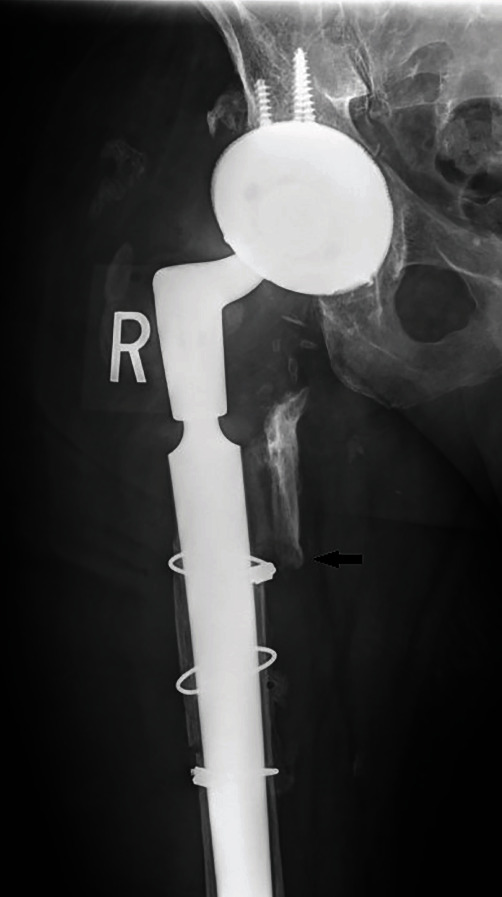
Anteroposterior X-ray of the right hip after reimplantation with fixation.
